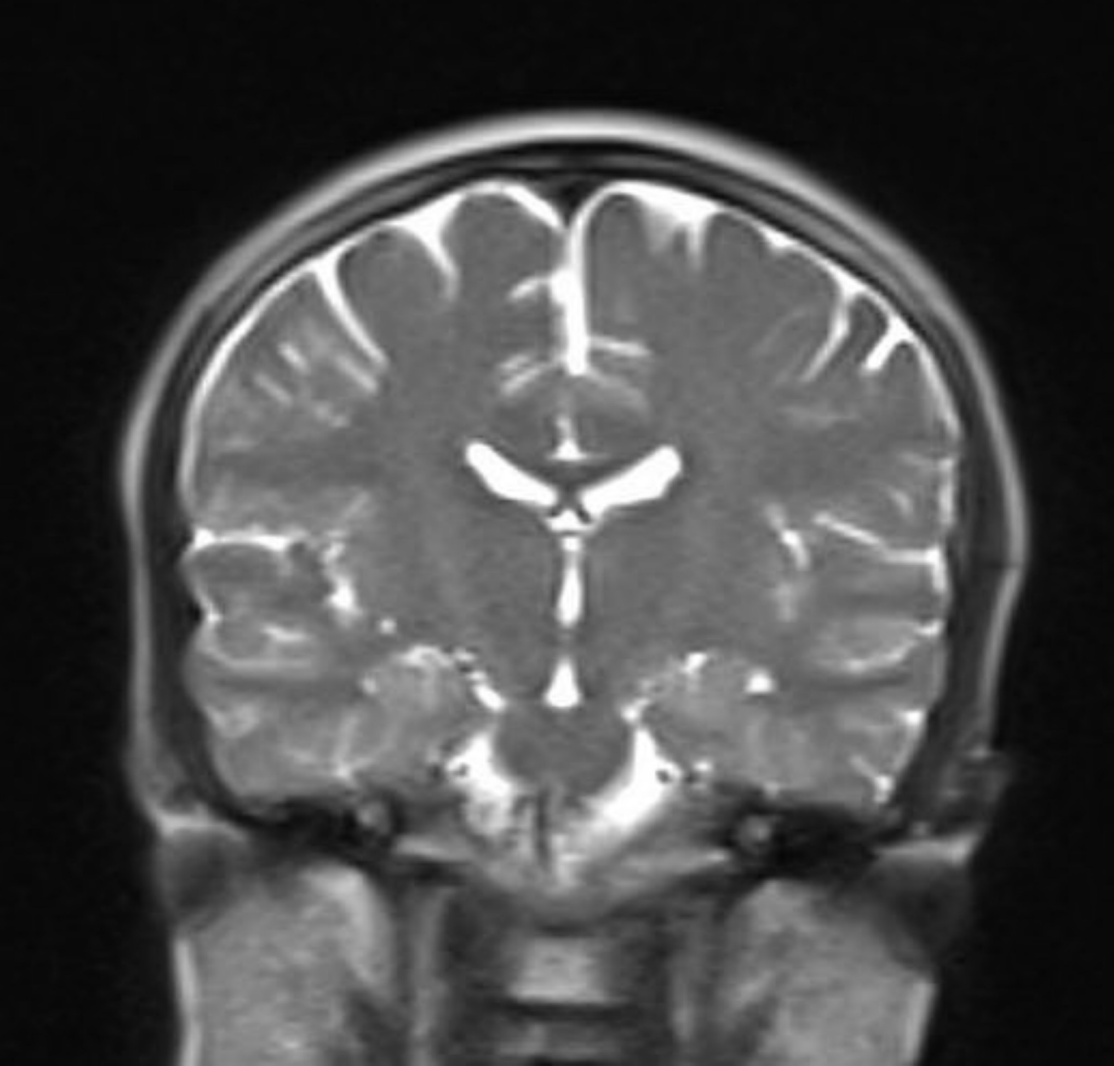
MRI of the brain: unremarkable study.
